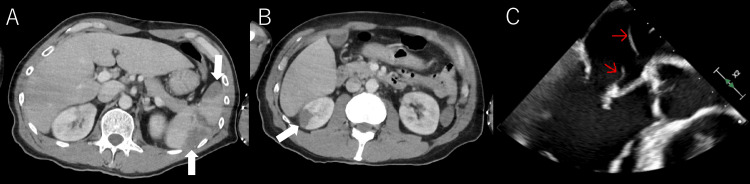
Abdominal CT and transthoracic echocardiography findings. (A, B): Contrast-enhanced abdominal CT reveals infarctions in the spleen and right kidney (white arrows). (C): Transthoracic echocardiography shows two threadlike to bandlike vegetations exceeding 30 mm attached to the anterior mitral leaflet (red arrows).
